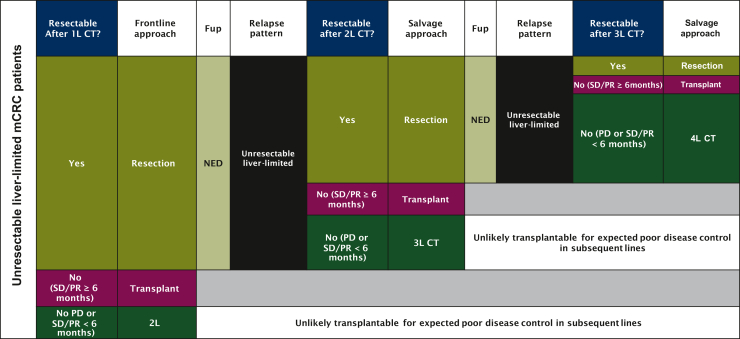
Real-world scenarios of application of liver transplantation in liver-limited mCRC. 1L, first-line; 2L, second-line; 3L, third-line; 4L, fourth-line; CT, chemotherapy; Fup, follow-up; mCRC, metastatic colorectal cancer; NED, no evidence of disease; PD, progressive disease; PR, partial response; QoL, quality of life; SD, stable disease.
